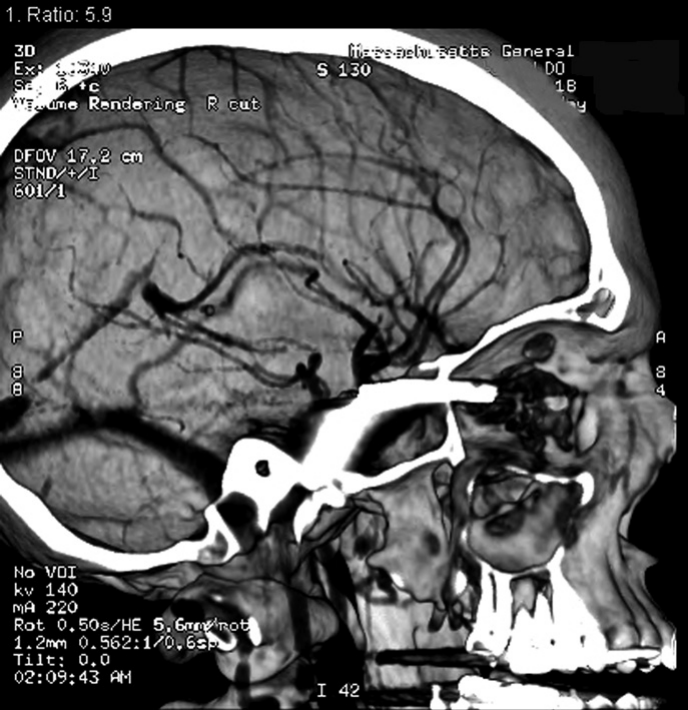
Sagittal contrast enhanced CTA scans demonstrating the retained metal fragment measuring as it is situated from the retro-orbital wall and curves through the temporal lobe into the skull base.
